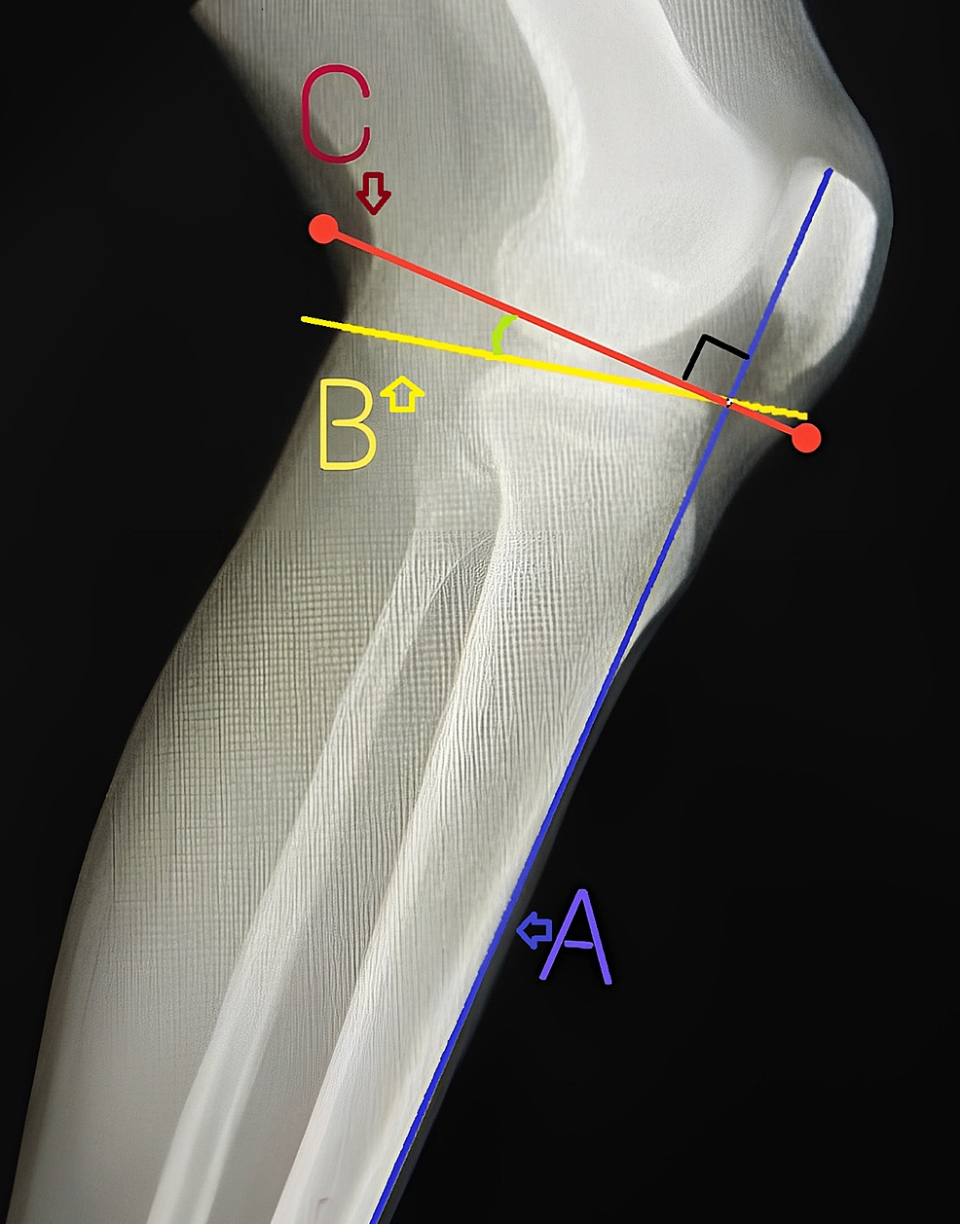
An X-ray of the knee joint (lateral view) showing the measurement of the posterior tibial slope (PTS) A: anterior cortical line; B: line tangential to the tibial plateau; C: line perpendicular to A at the point of intersection of A and B
